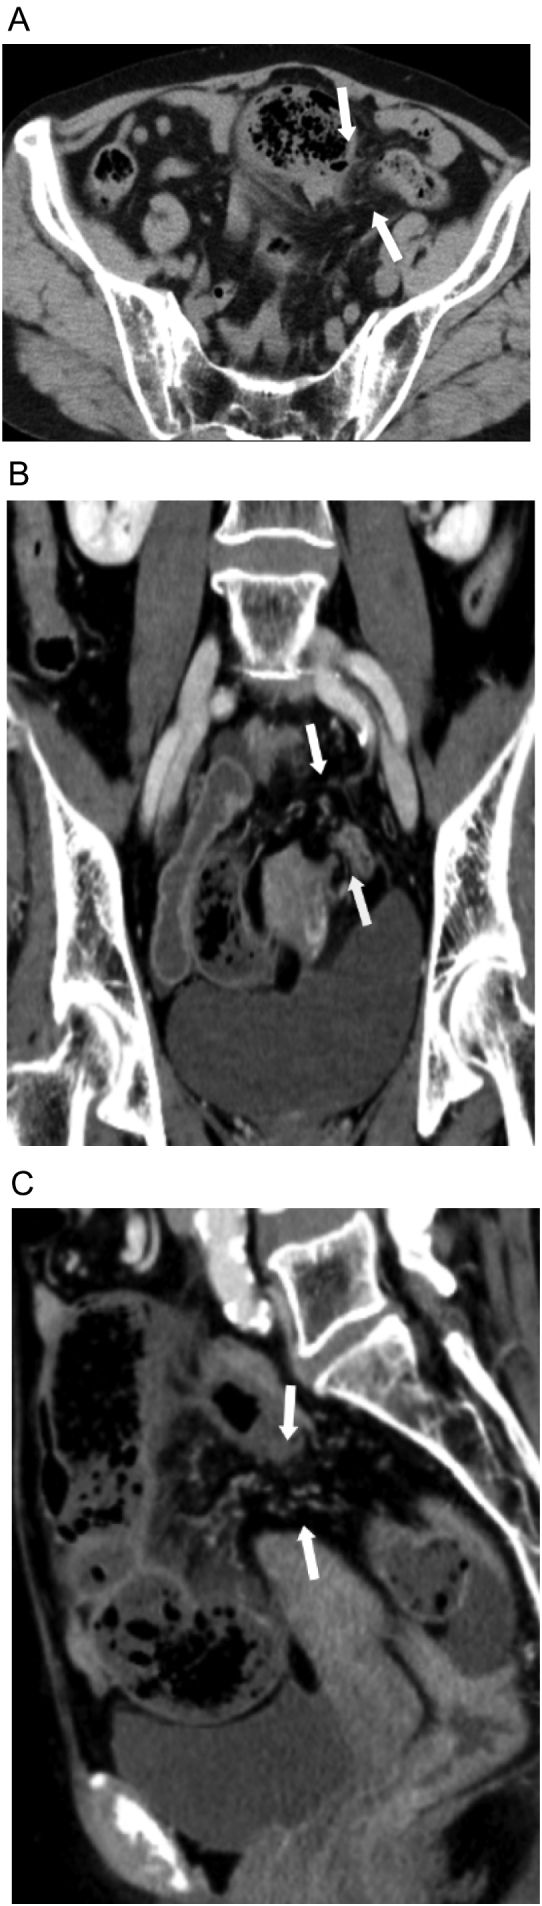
A computed tomography scan showing obstruction of sigmoid colon. Arrows show the defect of the broad ligament. A: Axial image: The uterus is compressed dorsally on the right, and the left ovary is compressed ventrally. B: Coronal image: Ascites collection is observed in Douglas' fossa. C: Sagittal image: Edematous mesentery of the sigmoid colon is observed in Douglas' fossa with ascites collection.
